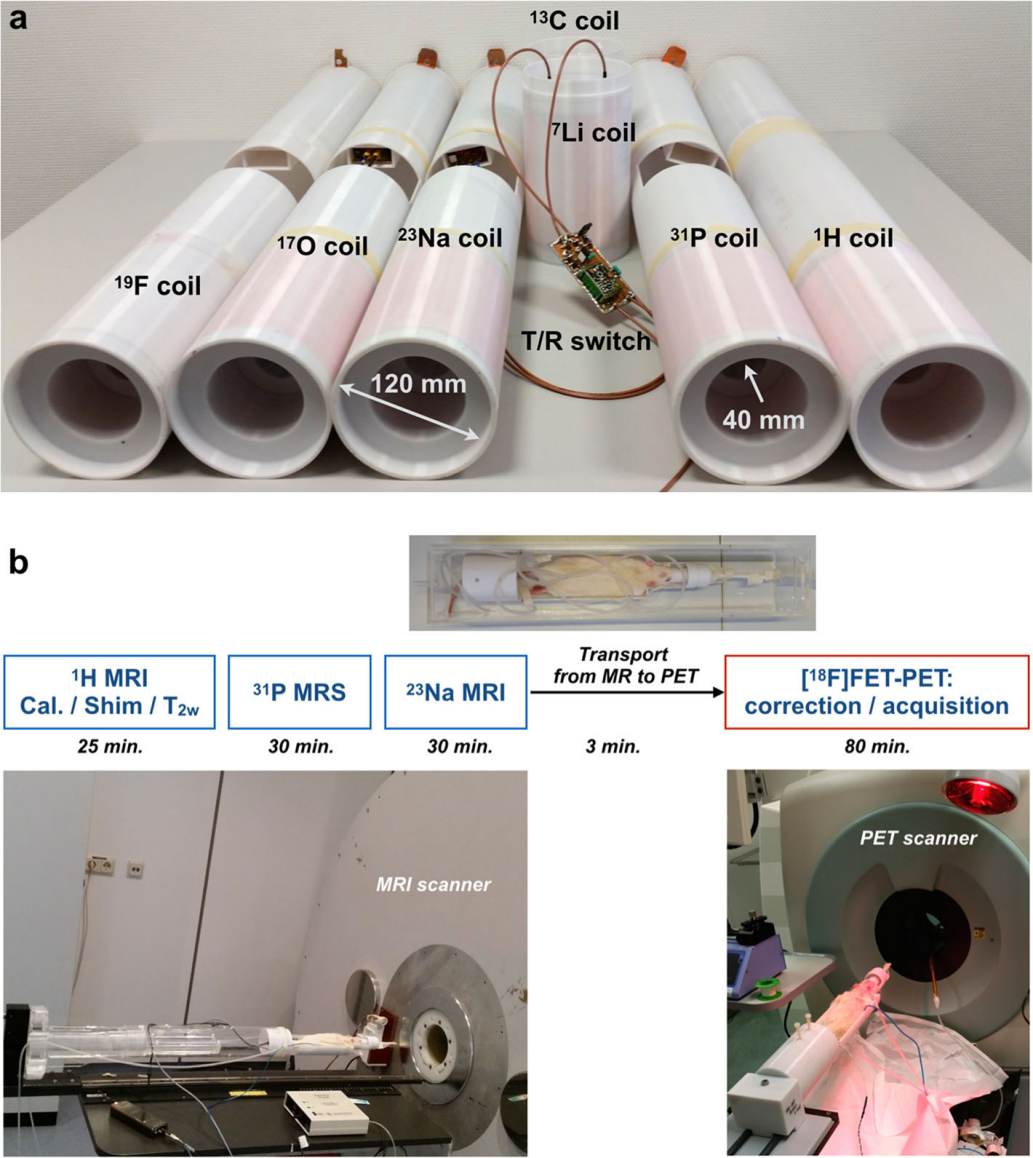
Picture of multinuclear MRI system showing RF coils and T/R switches (a), and MR, PET and transport systems with an example of measurement acquisition schemes (b)
